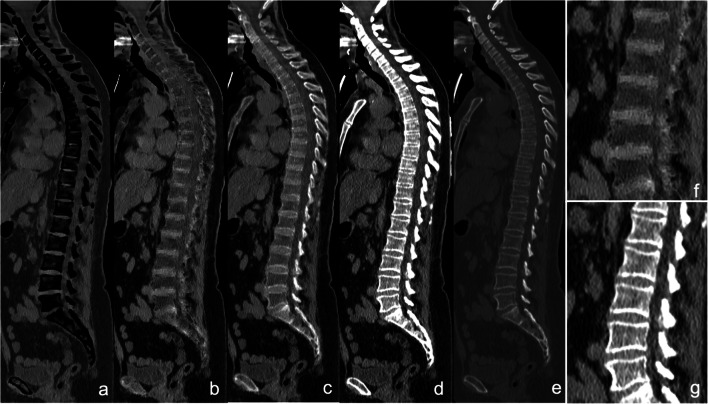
Patient with non-diffuse infiltration. a CaSupp index 25; b CaSupp index 65; c CaSupp index 95; d conventional CT soft tissue window; e conventional CT bone window; f, g magnification of b and d for L1-5
